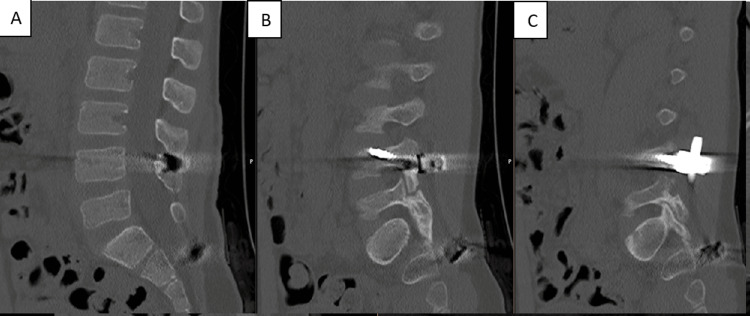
CT scan images at six months postoperatively (A) Sagittal view. (B)  Sagittal view-L4 screws. (C) Sagittal view-L4 screws
